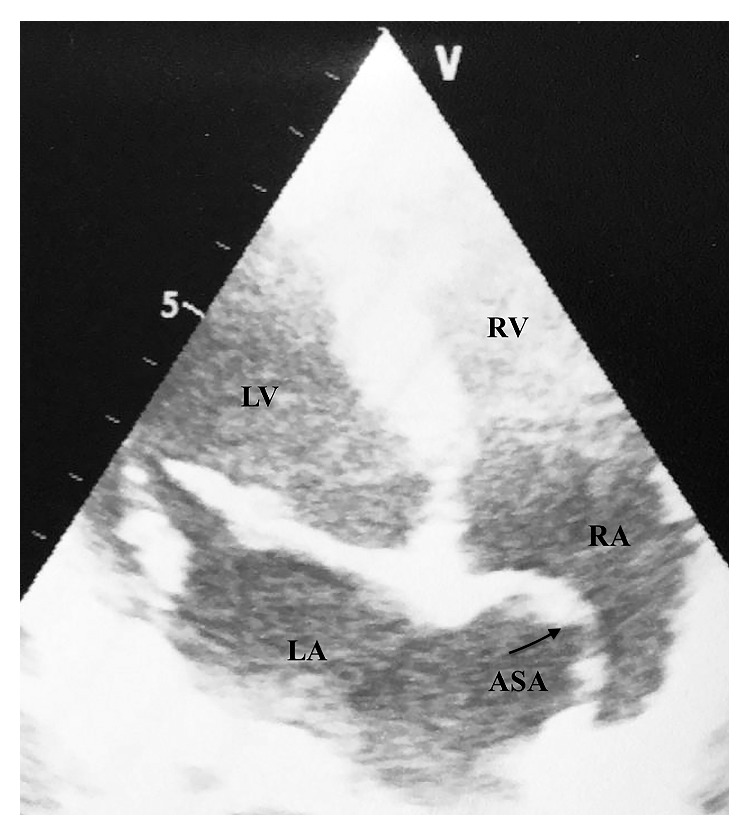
Apical four chamber view of atrial septal aneurysm detected by transthoracic echocardiography. ASA: atrial septal aneurysm, LV: left ventricle, LA: left atrium, RV: right ventricle, and RA: right atrium.
